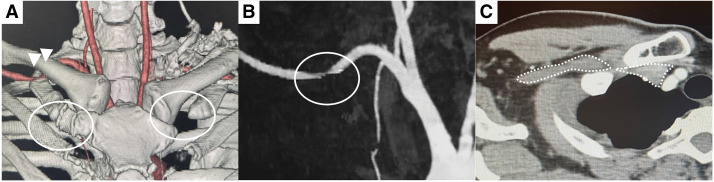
Computed tomography angiography in the arm-elevated position. (A, B) Asymmetry of the first ribs and stenosis of the subclavian artery at the costoclavicular space. (C) Stenosis of the subclavian vein at the costoclavicular space.
